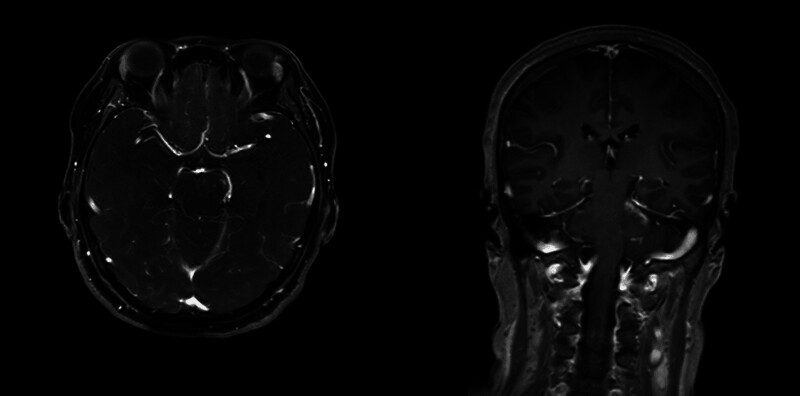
Brain MRI enhancement reveals abnormal enhancement foci in the bilateral pontine brachium, pons and soft meninges.
